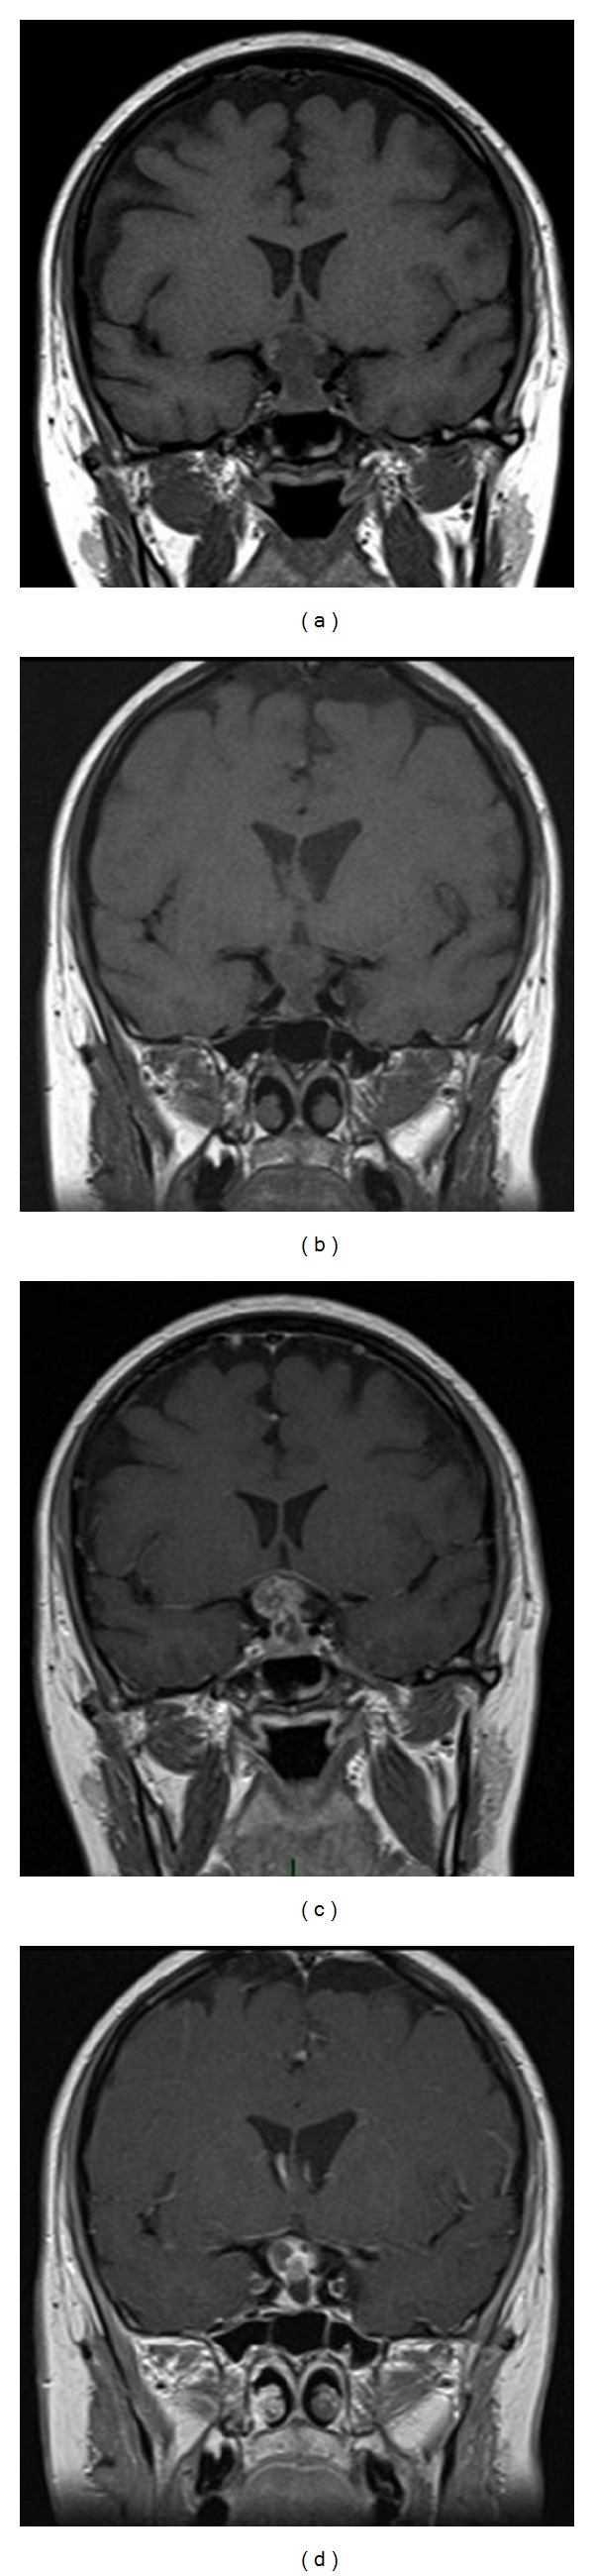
Coronal preoperative ((a) without gadolinium; (c) with gadolinium) and postoperative ((b) without gadolinium; (d) with gadolinium) T1 MRI.
